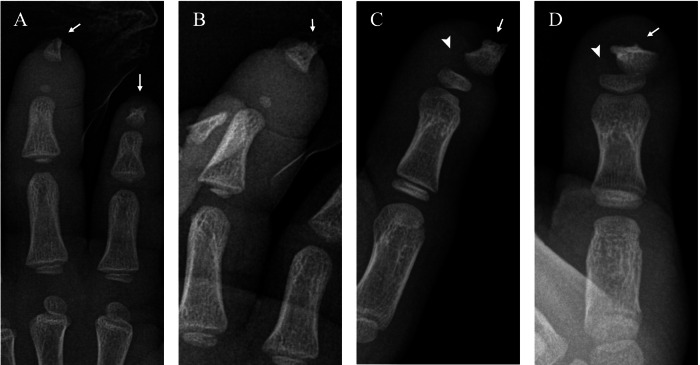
Acro-osteolysis in the left hand of a patient with hereditary sensory and autonomic neuropathy type IV at 2 (A, B) and 8 years of age (C, D). AP (A, D) and lateral (B, C) radiographs show bone resorption (arrows) of the distal phalanges of the 2nd and 3rd (A, B) and the 1st (C, D) fingers; note the epiphyseal separation (arrowhead) of the distal phalanx
